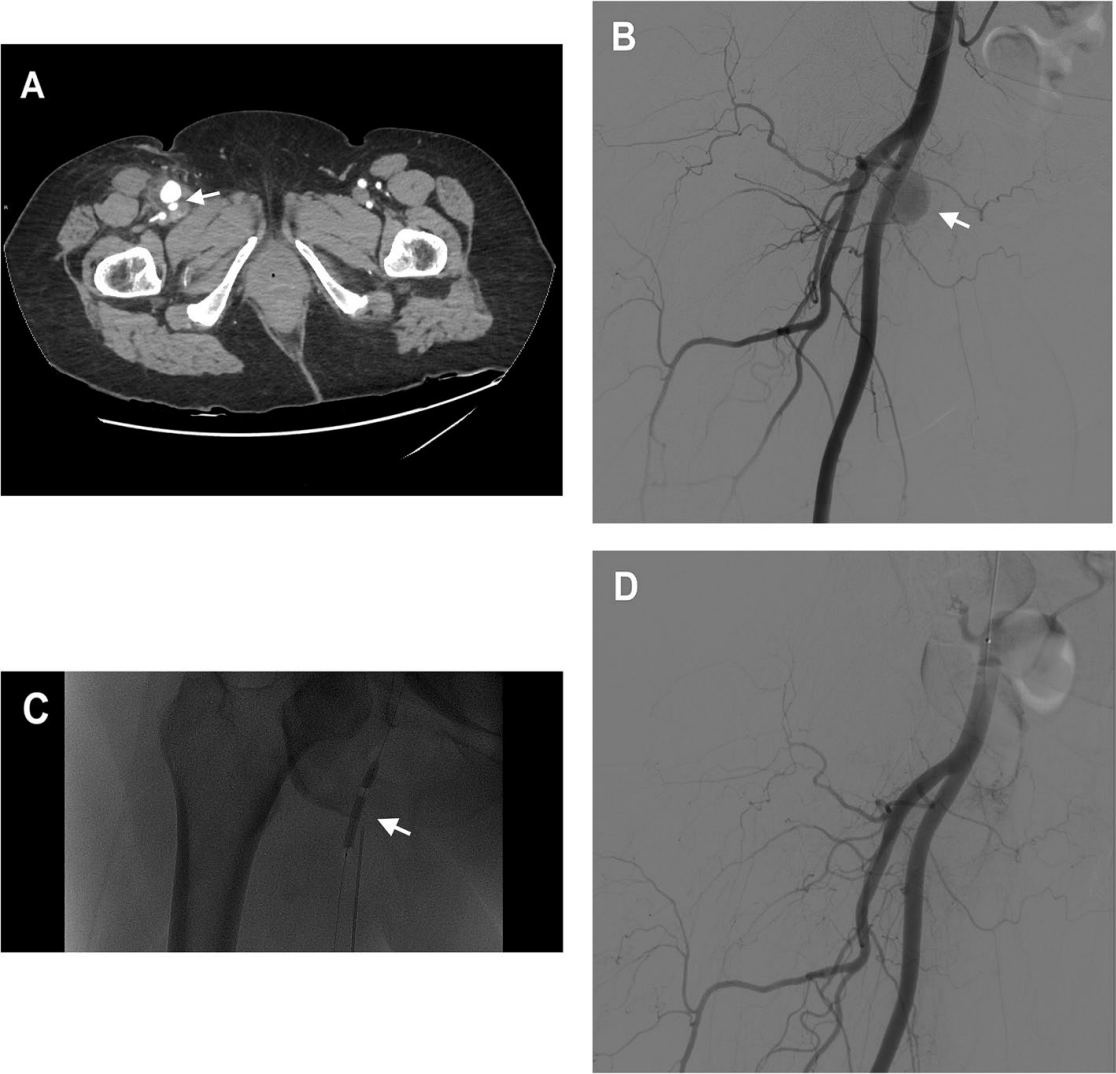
A 71 years-old patient who suffered from a post-catheterization pseudoaneurysm of the right SFA with a short and wide neck, measuring 30 × 26 mm. a Contrast-enhanced CT scan in the arterial phase showing the SFA pseudoaneurysm (arrow) b Digital subtraction angiography (DSA) of the common femoral artery using contralateral femoral access showing the large pseudoaneurysm of the SFA (arrow) c procedural DSA showing balloon occlusion of the neck of the pseudoaneurysm while performing percutaneous thrombin injection (arrow) and d post-procedural DSA showing absence of contrast uptake by the pseudoaneurysmal sack with normal opacization of the CFA
